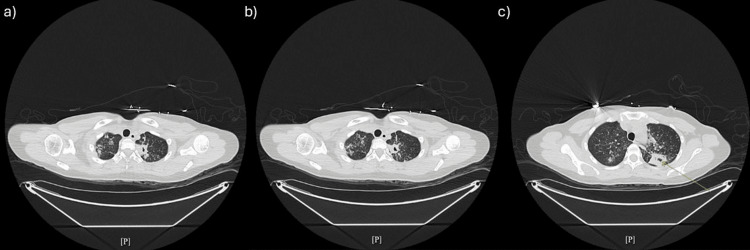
Dual-phase CT angiogram of the chest. (a) Axial CT slice demonstrating bilateral tree-in-bud micronodularity and cavitary changes. (b) Axial slice demonstrating necrotizing consolidation in the left upper lobe adjacent to segmental pulmonary arterial branches. (c) Contrast-enhanced image demonstrating the peribronchial consolidation with internal air foci and peripheral enhancement consistent with the likely bleeding source.
